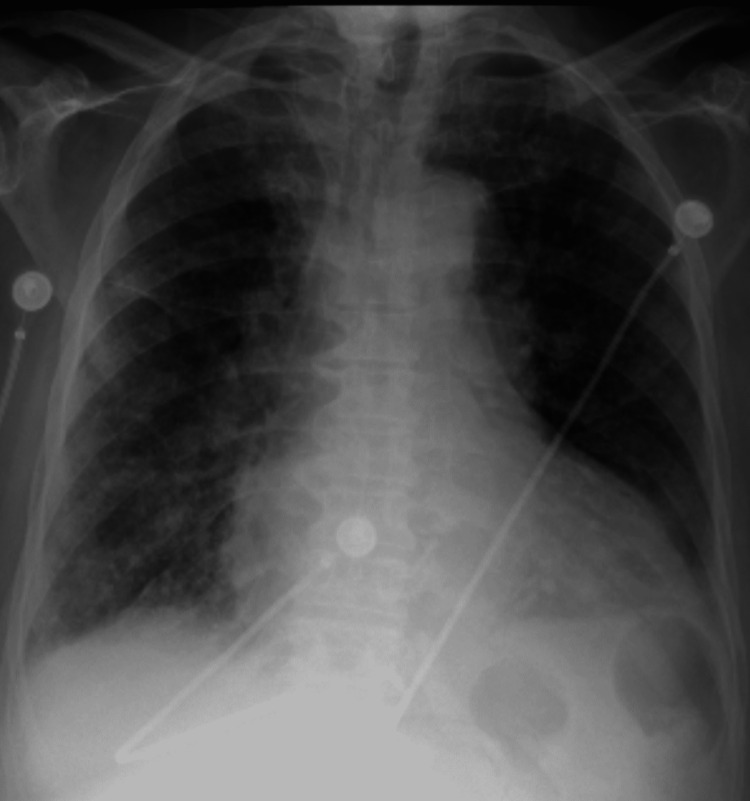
Admission chest radiography with no pneumothorax
